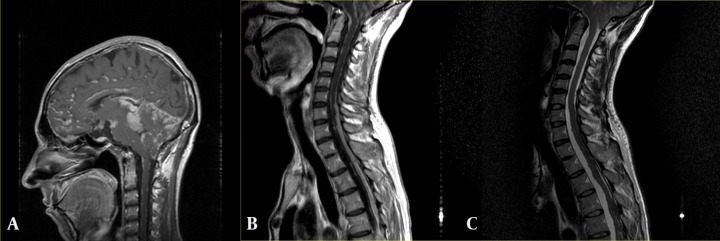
A, Brain mid-sagittal contrast enhanced T1-weighted image clearly depicts leptomeningeal lesions in the posterior fossa and resultant downward tonsillar herniation; B, Similar diffuse enhancing leptomeningeal lesions are notable in contrast enhanced T1 mid sagittal image through the cervical and upper thoracic spine. Tonsillar herniation is also visible in the top of this image. (C) Sagittal T2-weighted image through cervical and upper thoracic spine better delineates tonsillar herniation.
